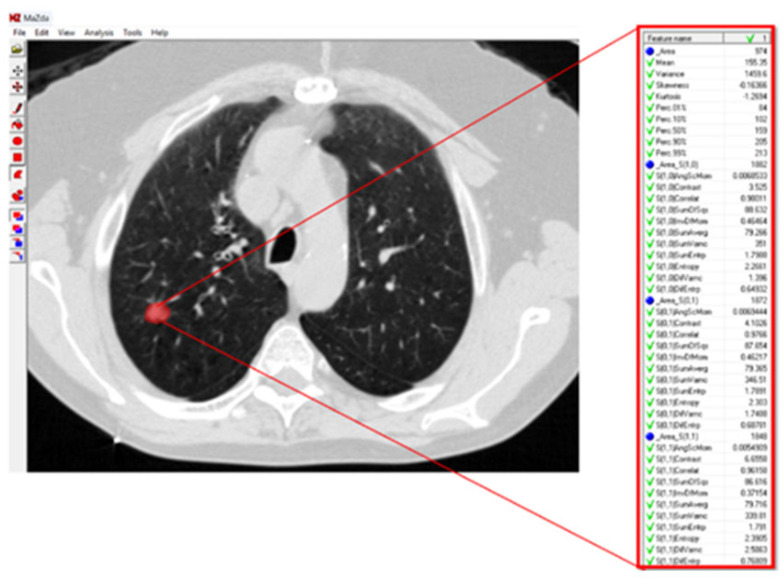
Lung CT scan with GGO texture analysis. About one-third of the variables extracted by the MaZda 4.0 software for the texture analysis of the GGO in this lung CT scan are presented. The red circle denotes the GGO region of interest.
